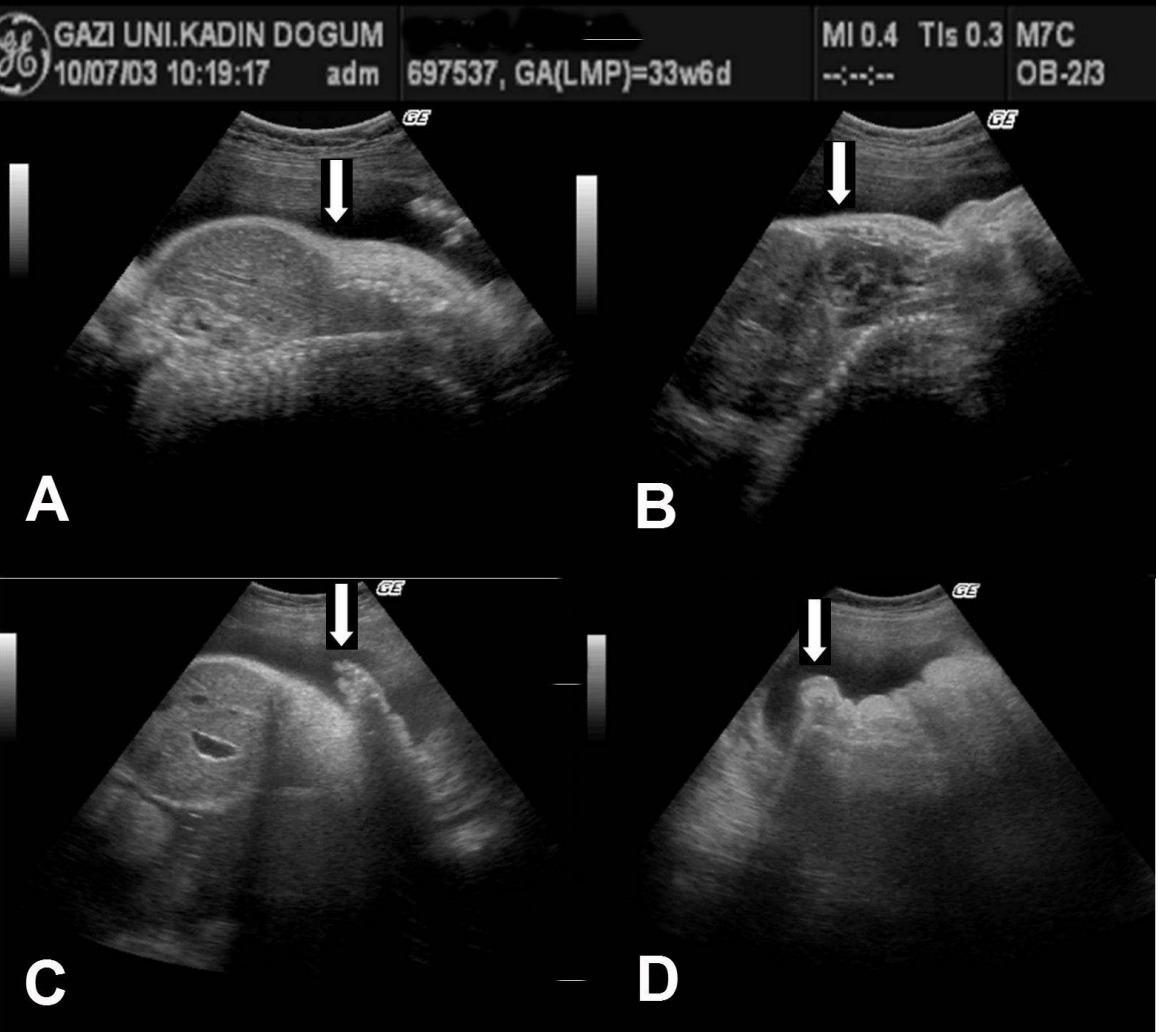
High abdomen/thorax ratio (A), pulmonary hypoplasia and narrow thorax (B), extreme micromelia (C, D) are observed in the prenatal sonogram at 33 weeks and 6 days of gestation.
